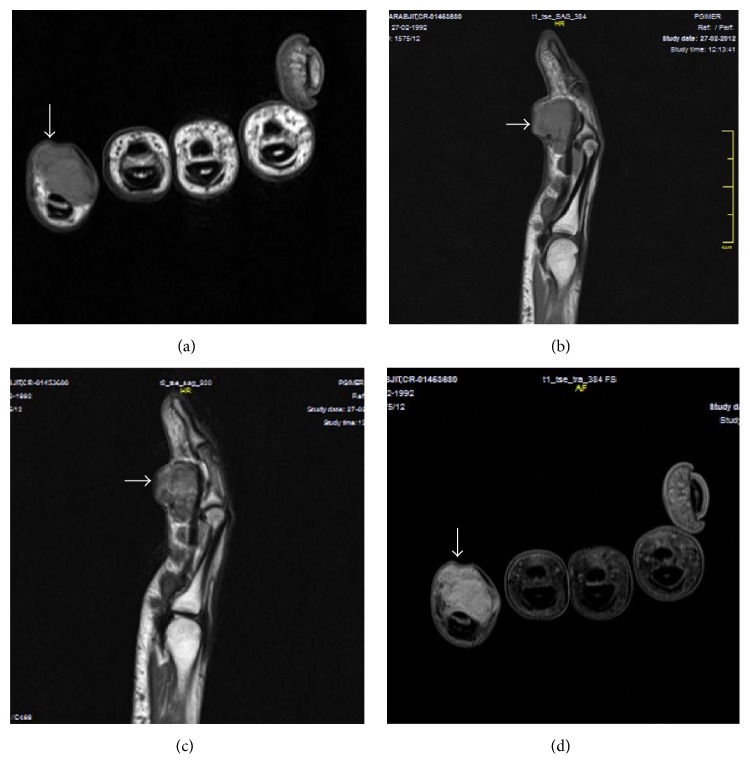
Giant cell tumor of tendon sheath. MRI (of the same patient as in Figure 3) show multiple, well-defined, lobulated, T1W, and T2W hypointense lesions ((a)–(c)) along the flexor tendons of right 5th finger. These lesions show mild enhancement seen on postgadolinium T1FS images (d).
